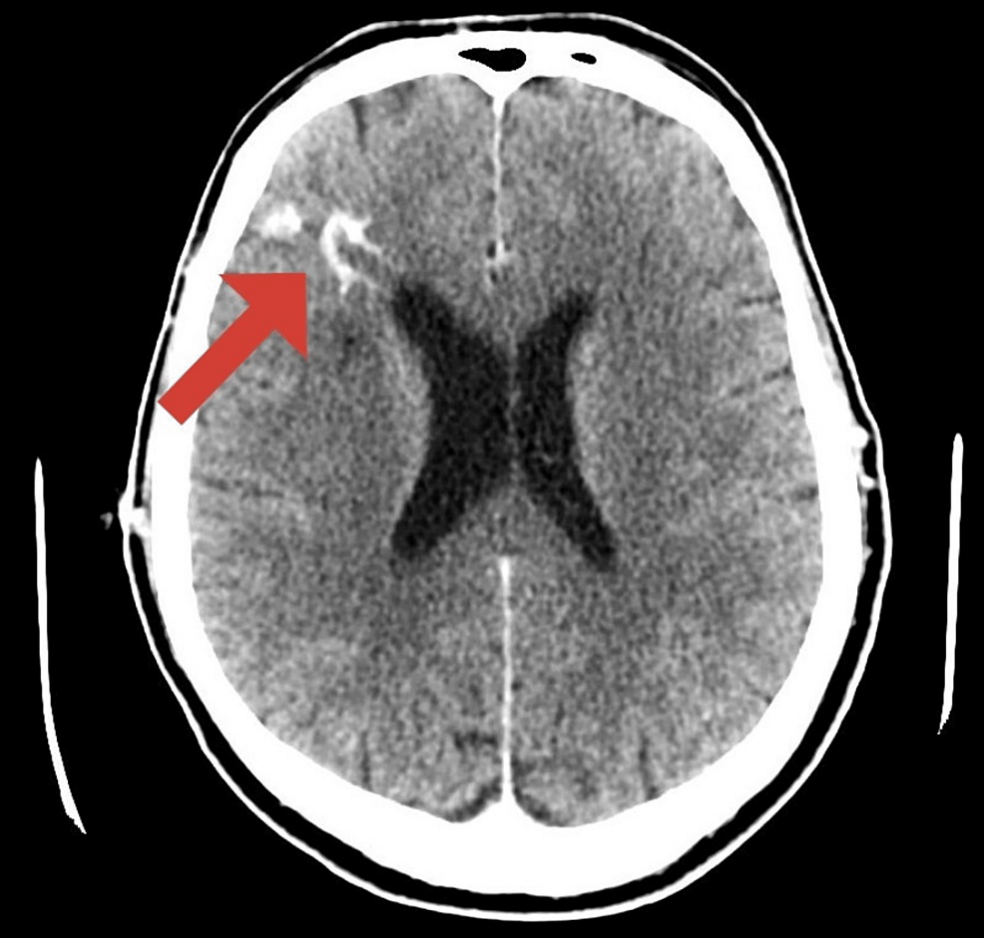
CT Brain with contrast shows a hyperdense area with an abnormal tuft of vessels in the right frontal cortex.
